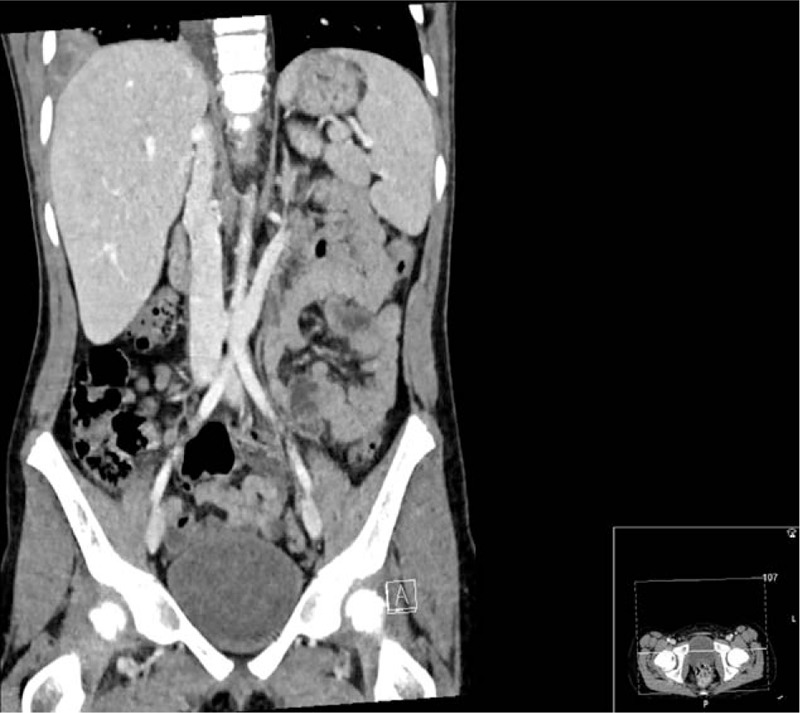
Coronal slice of computed tomography scan of abdomen and pelvis showing right lower lobe consolidation.
